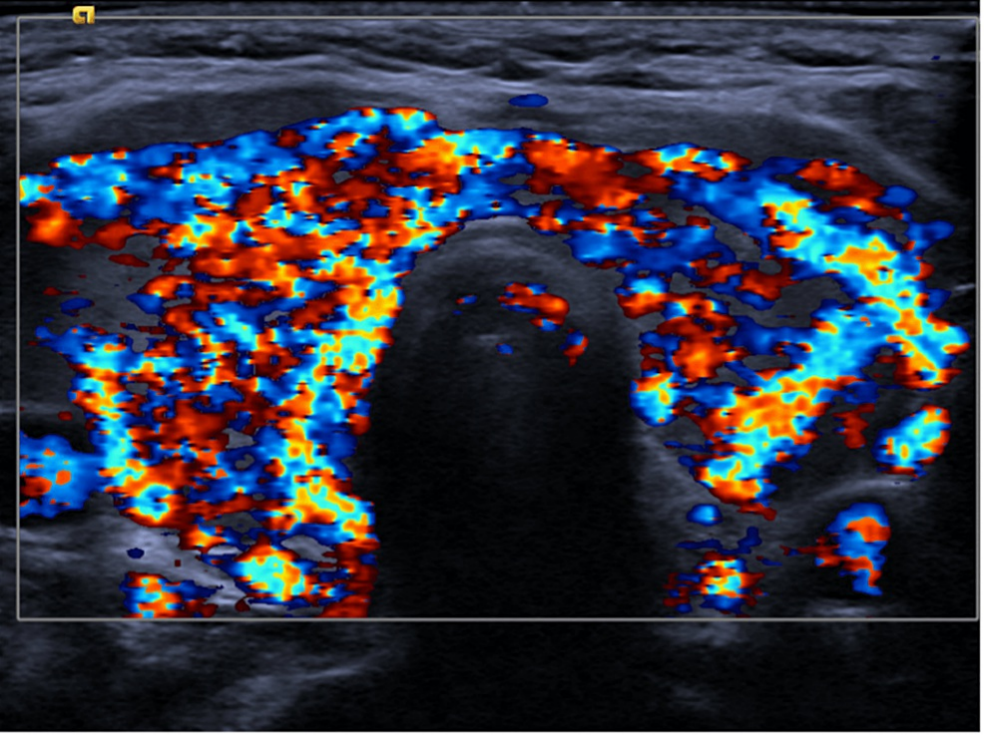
Ultrasound of the thyroid gland with Doppler Ultrasound of the thyroid gland showing increased vascularity on color Doppler consistent with thyroid inferno.
